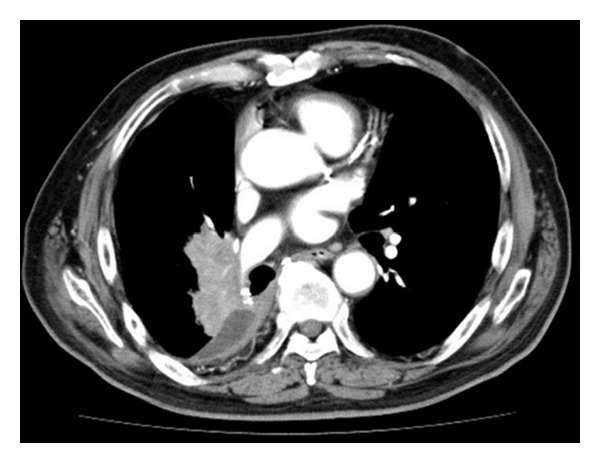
Computed tomography (CT) scan of the chest shows a mass opacity involving the right hilar lymph node.
